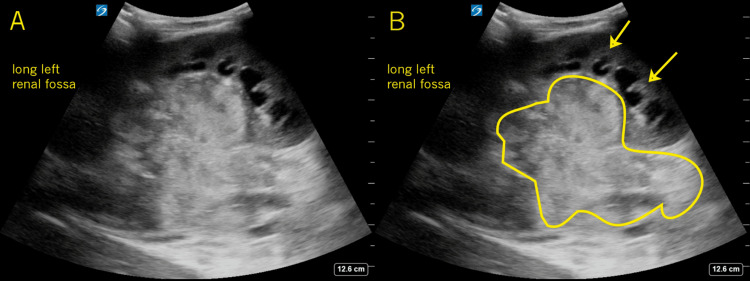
Long Left Renal Fossa In this longitudinal image from the left renal fossa, the enlarged kidney is seen (frame B arrows) with some dilation of the renal pyramids. Additionally, a large echogenic mass involving the entire renal pelvis is seen (frame B outlined). These findings should be recognized as abnormal to anyone with a basic understanding of normal sonographic anatomy.
